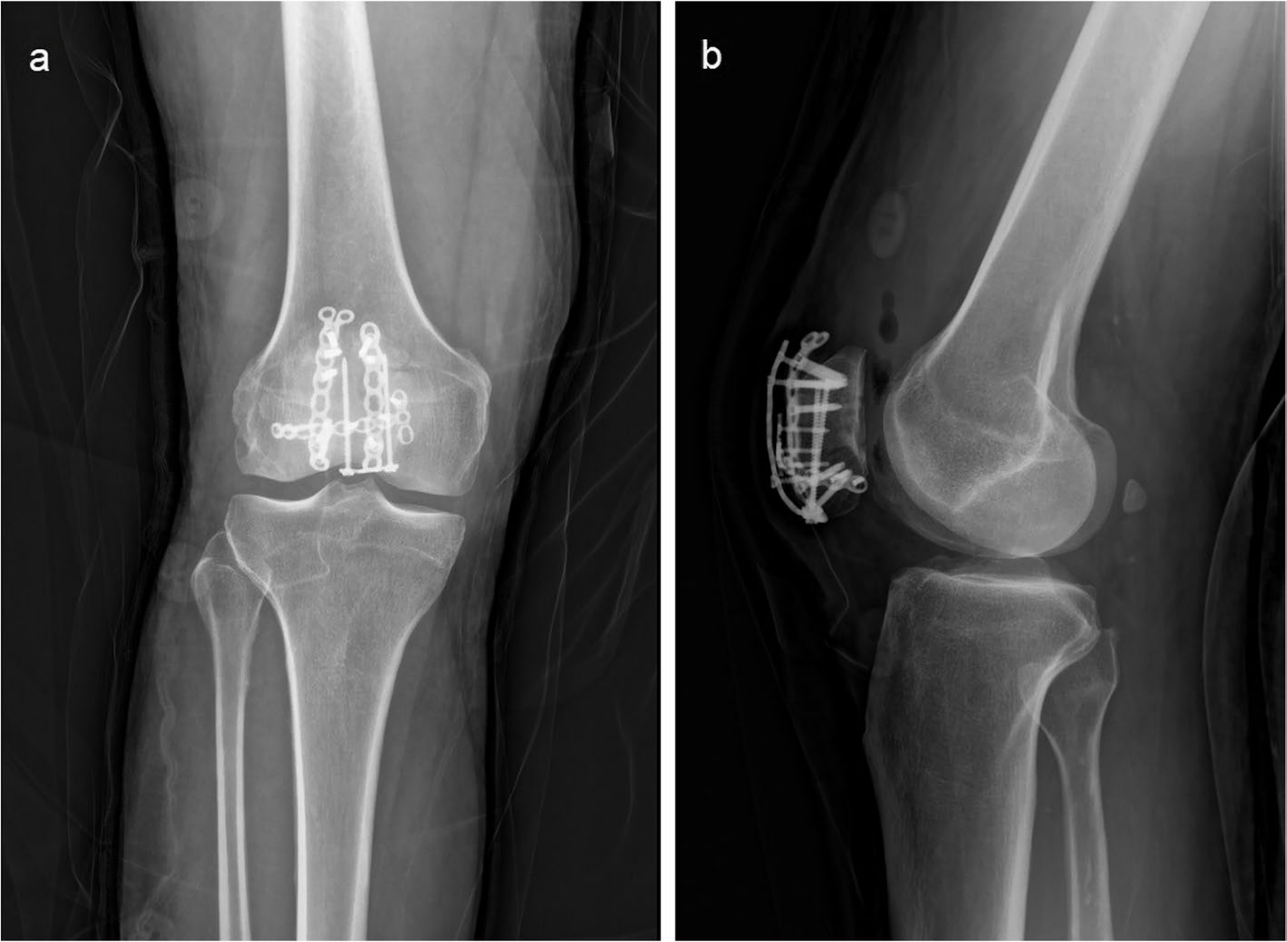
Postoperative simple radiograph of Case 1 a AP view b lateral view
